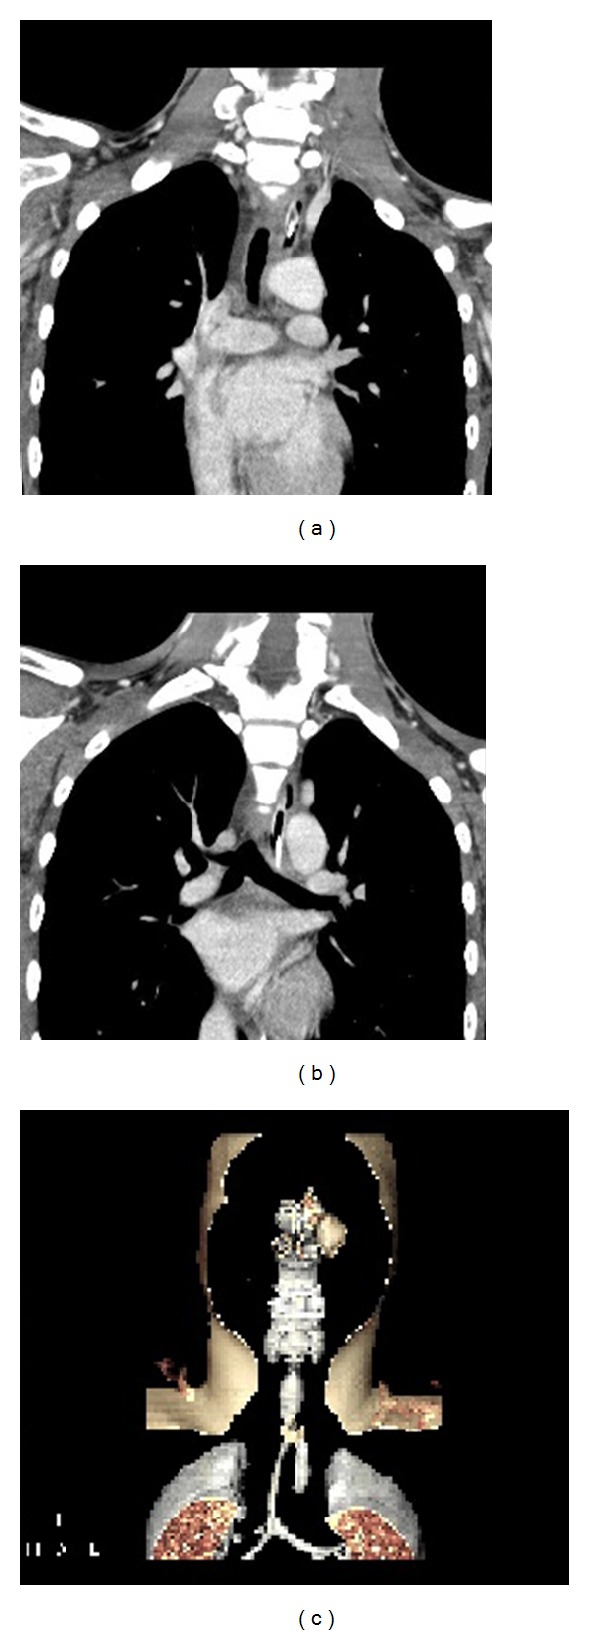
Initial CT of airway in coronal view with narrowed tracheal lumen of 1 cm (a) and congenital loss of right main bronchus (b), reconstructed in 3D (c).
